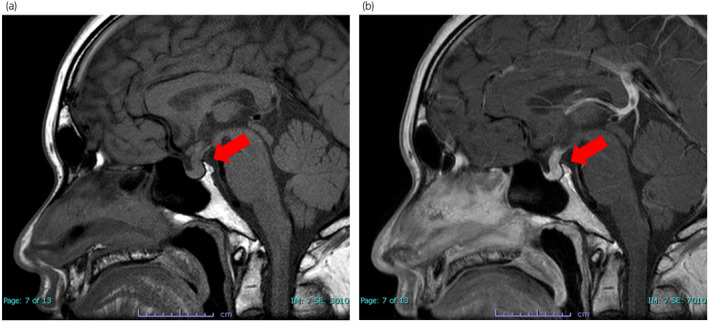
Radiological findings. (a) MRI T1‐weighted images reveals enlargement of the pituitary stem and no pituitary tumors. (b) MRI T1‐weighted images reveals uniformly enhanced pituitary stem, and lymphocytic hypophysitis is suspected.
